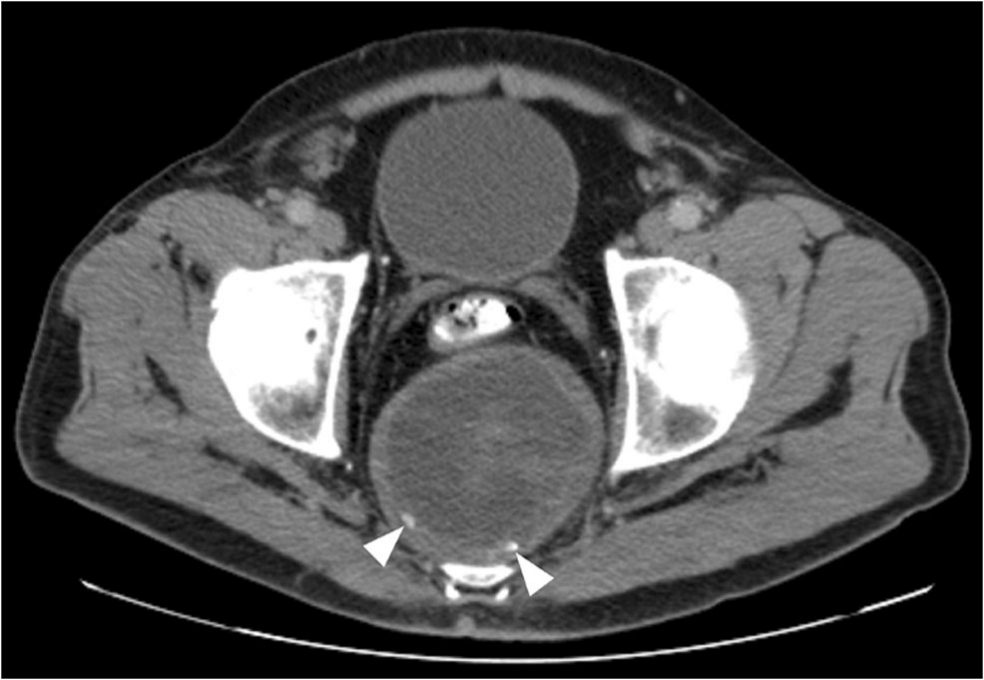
A 69-year-old man with ancient schwannoma in the presacral space. Axial CT shows a well-defined mass with hypodense center, enhancing capsule, and peripheral calcifications (*arrowheads*) in the presacral space. Pathological analysis after biopsy confirmed ancient cystic schwannoma. The mass remained unchanged after 10 years’ follow-up
